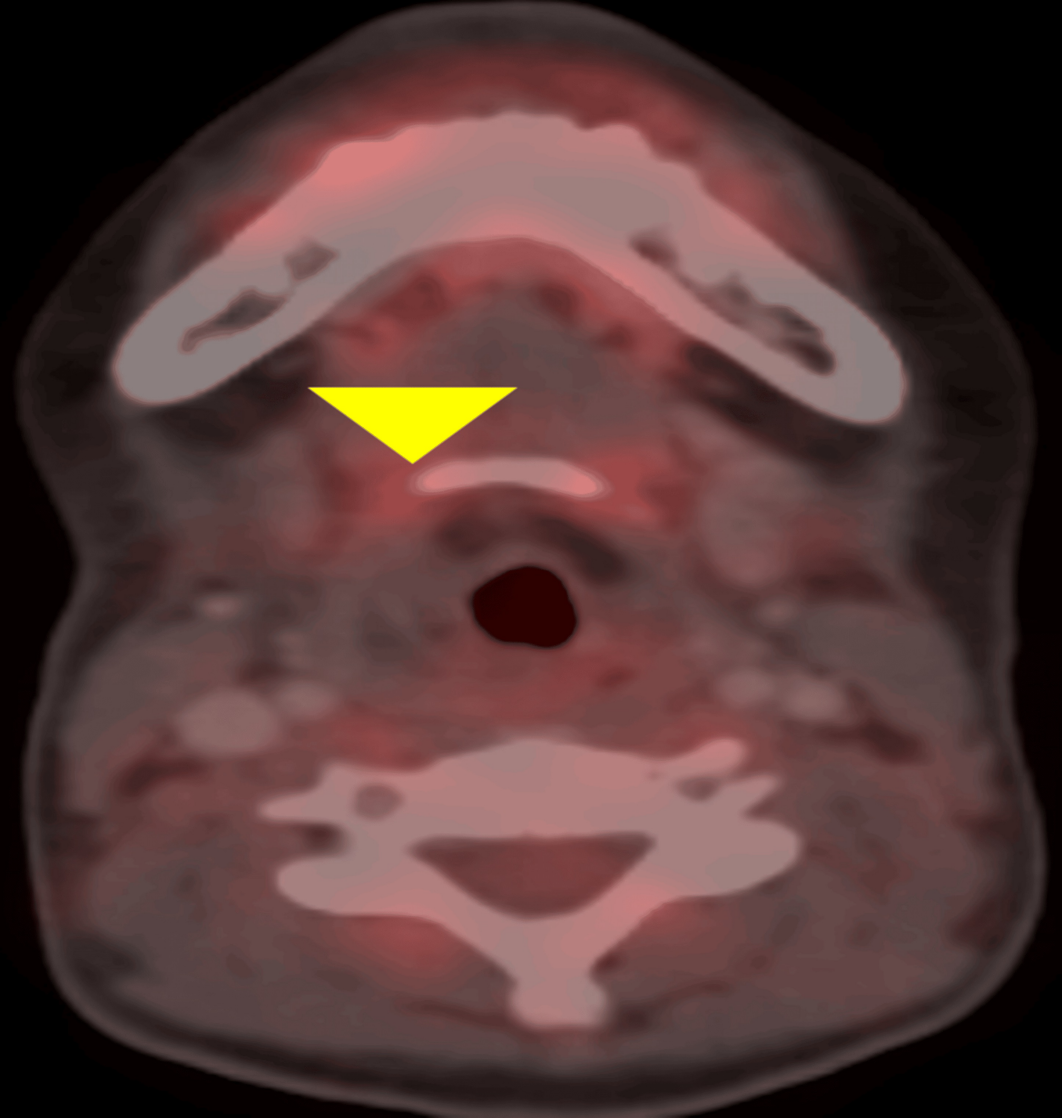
Yellow arrowhead showing the previous growth site; now, no growth in the PET/CT image post chemotherapy and post radiotherapy; complete metabolic resolution. PET/CT: positron emission tomography/computed tomography
